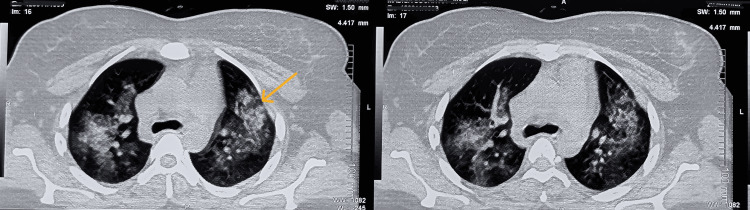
Post-treatment HRCT thorax showing resolution of hemorrhage. Near-complete resolution of DAH following pulse steroids and five cycles of plasmapheresis. DAH: diffuse alveolar hemorrhage
